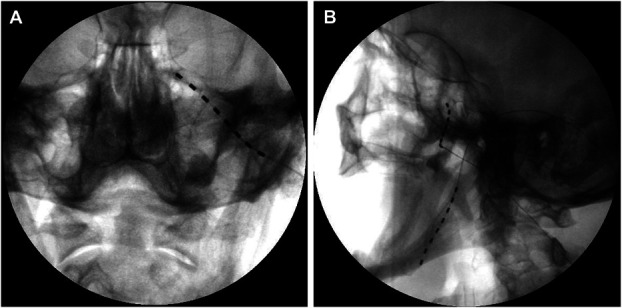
X-ray images showing sphenopalatine ganglion at Anterior-Posterior view (A) and sphenopalatine ganglion + V3 at lateral view (B) neuromodulation leads position.
